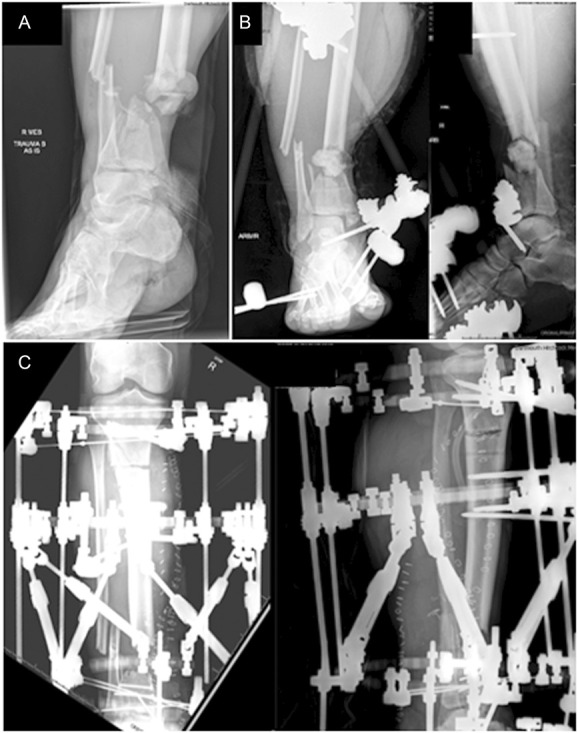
Injury radiograph (A), initial postdebridement radiographs (B), and radiographs after frame placement with proximal tibial osteotomy for distraction osteogenesis/bone transport (C).
